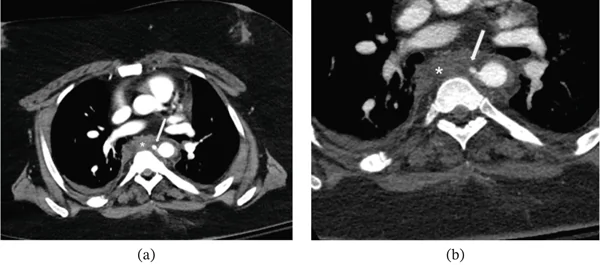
(a and b) Axial computed tomography angiography of the chest demonstrates a prevertebral hematoma at the level of T6 ( ^∗^). Image 2b represents a slightly zoomed‐in view to better visualize the 6‐mm contrast‐filled outpouching arising from the right lateral aspect of the descending thoracic aorta (←).
